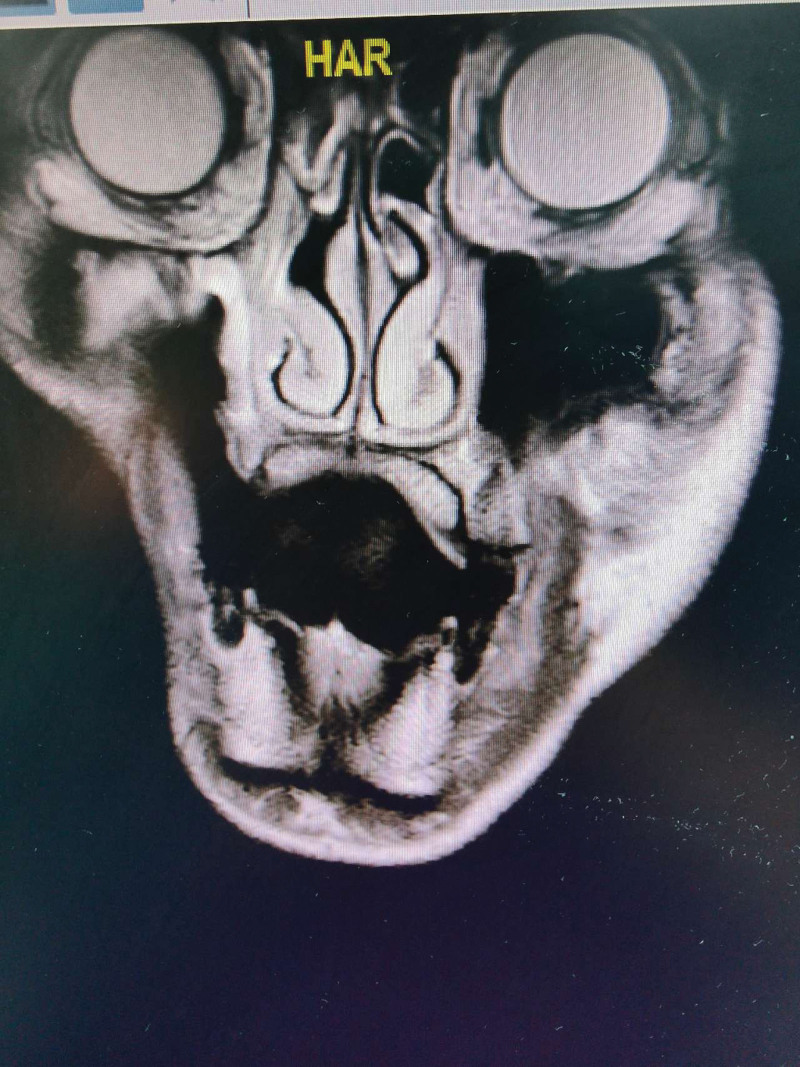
CT showing the patient’s right maxillary resection and anatomical changes of the upper airway. CT = computed tomography.
